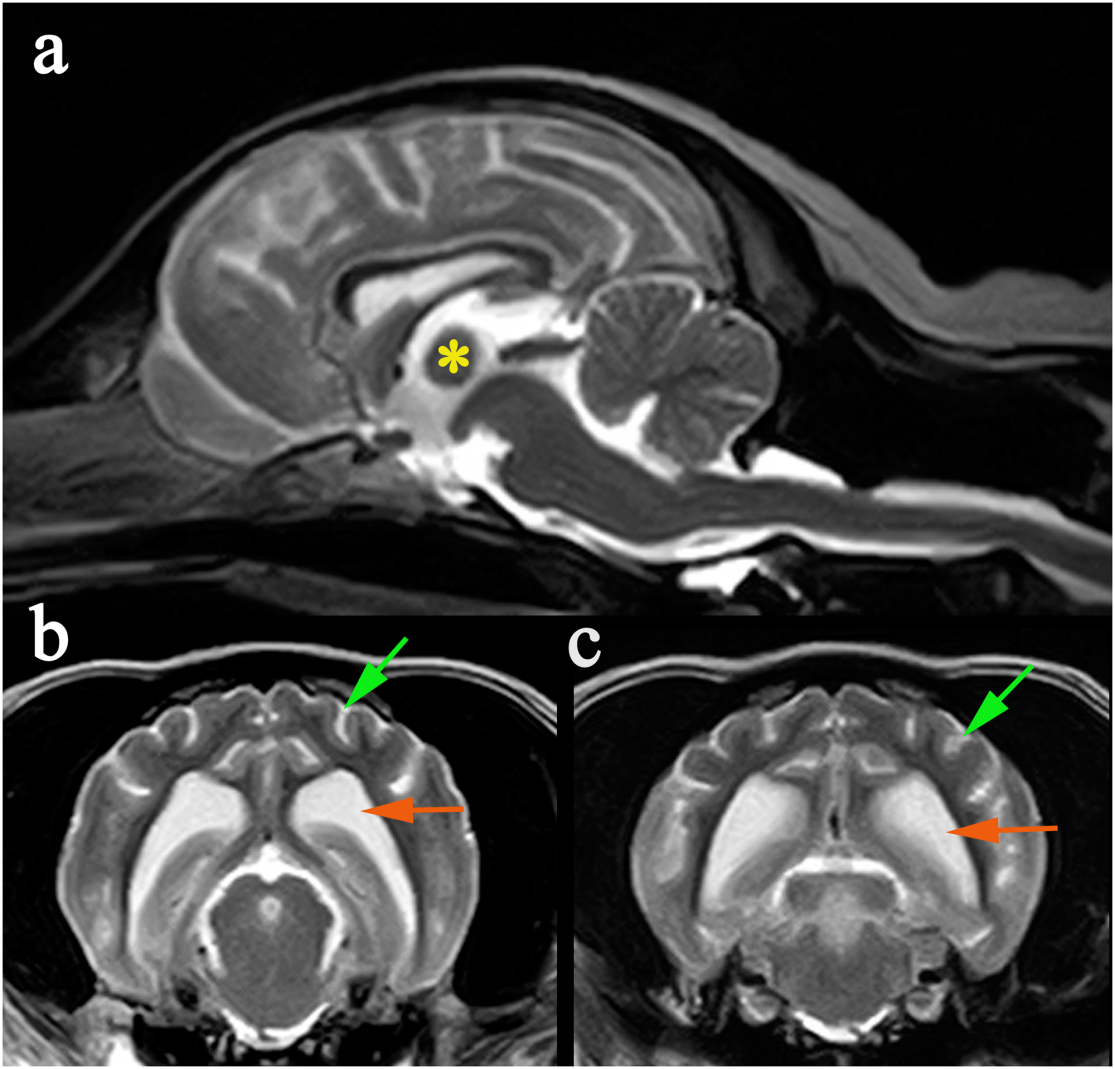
MRI changes in an 8 year old male neutered MWHD with advanced LD. (a) Mid-sagittal T2W image of the brain demonstrating atrophy of the intra-thalamic adhesion (*). (b) Transverse T2W at the level of temporal lobes demonstrating cortical atrophy with widening of the subarachnoid space (green arrow) and enlargement of the lateral ventricle (orange arrow). (c) Transverse T2W at level of occipital lobes demonstrating cortical atrophy with widening of the subarachnoid space (green arrow) and enlargement of the lateral ventricle (orange arrow).
